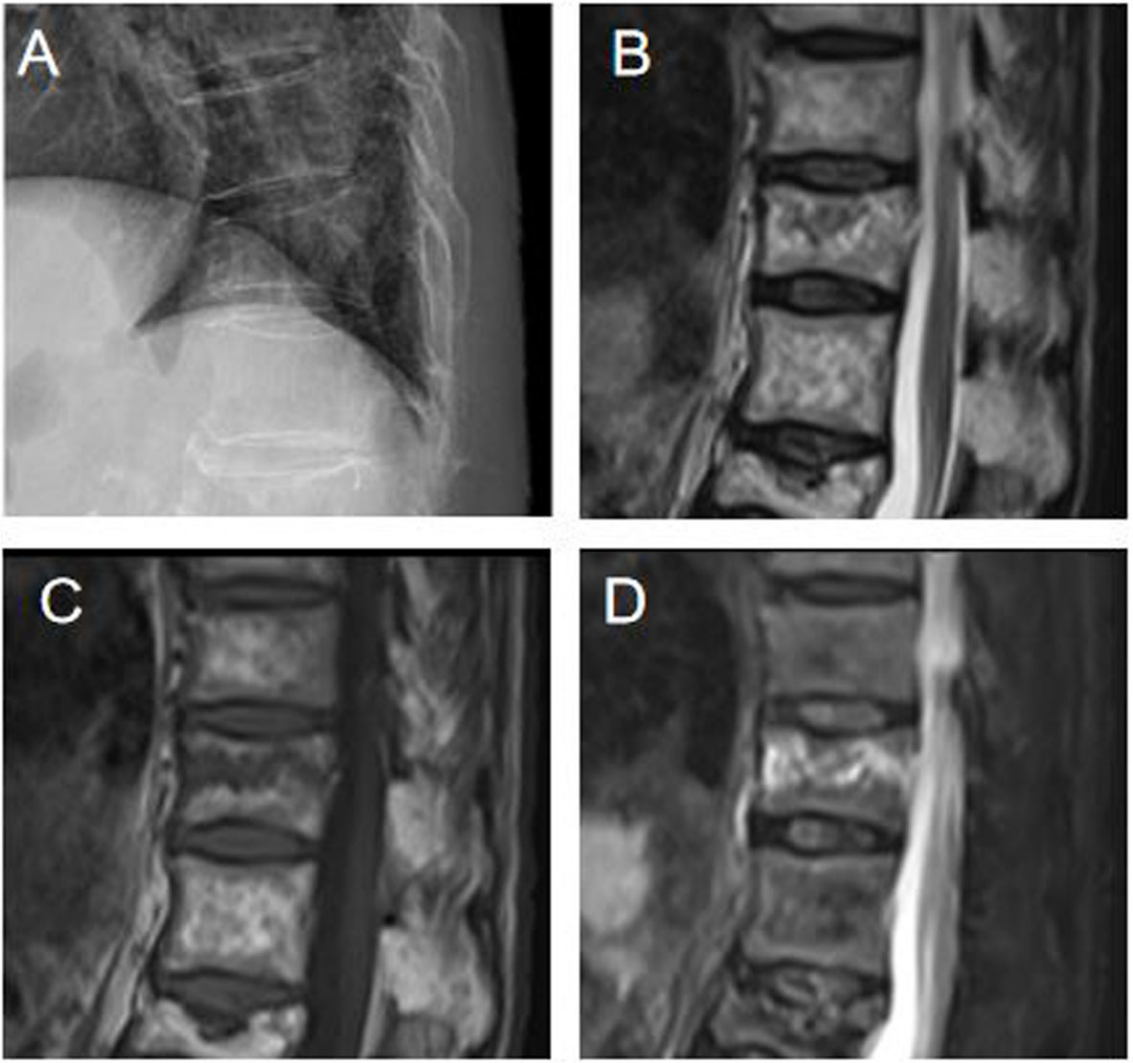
An 84-year-old female patient visited for lumbago after she slipped, and there was a T11 vertebral body fracture based on the lateral plain radiograph (A). A recent fracture in T2WI (B), T1WI (C), and fat suppression image (D) was confirmed based on the MRI
